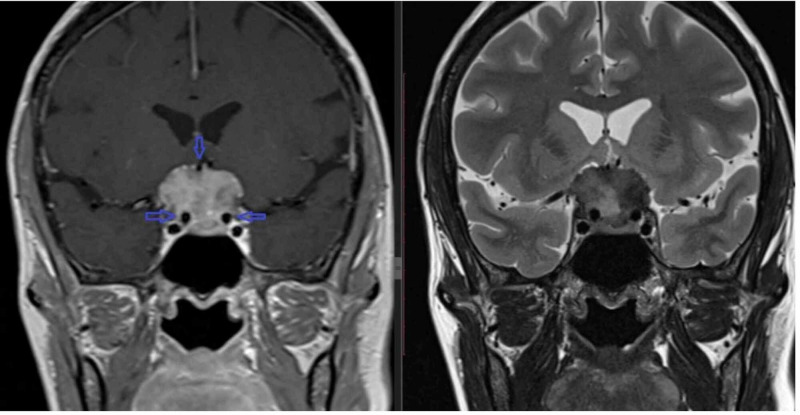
T1-weighted post-contrast shows intensely enhancing sellar and suprasellar mass, with encasement of the supraclinoid part of bilateral ICA (blue arrows). There is the encasement of the right anterior cerebral artery (A1 segment). T2-weighted coronal sequences show mass lesion returning isointense signals similar to gray matter. ICA: internal carotid artery
